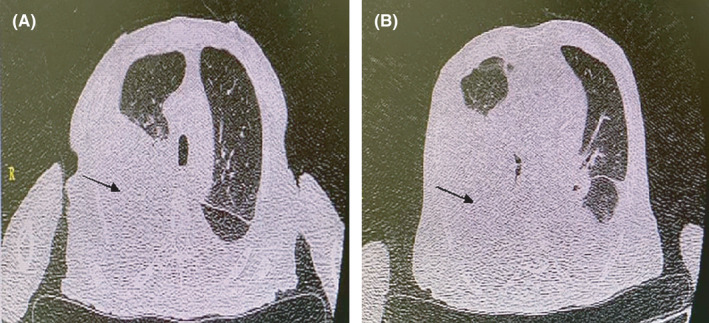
A‐B, HRCT chest imaging. A moderate volume of pleural effusion in the left lung, a large volume with a collapse of the lower lung on the right, and complete collapse of the lower right lobe of the right lung. A, On the third day of hospitalization and after three days of treatment. B, On the first day of hospitalization
